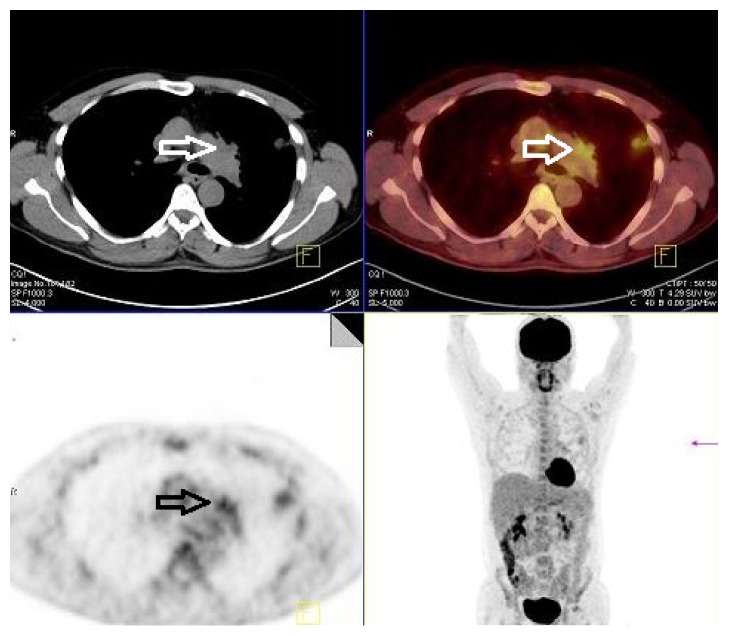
First PET/CT: axial CT, pet, fused PET/CT, and coronal MIP images revealed a hypermetabolic mass (arrows) in the left aortopulmonary region and hypermetabolic nodule in the anterior segment of the upper lobe of the left lung.
